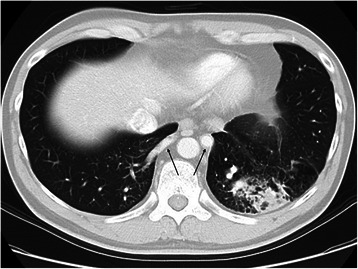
Computed tomography (CT) of the chest showed consolidation of the left lower lobe with no confined sequestrated area and two aberrant arteries (arrows)
